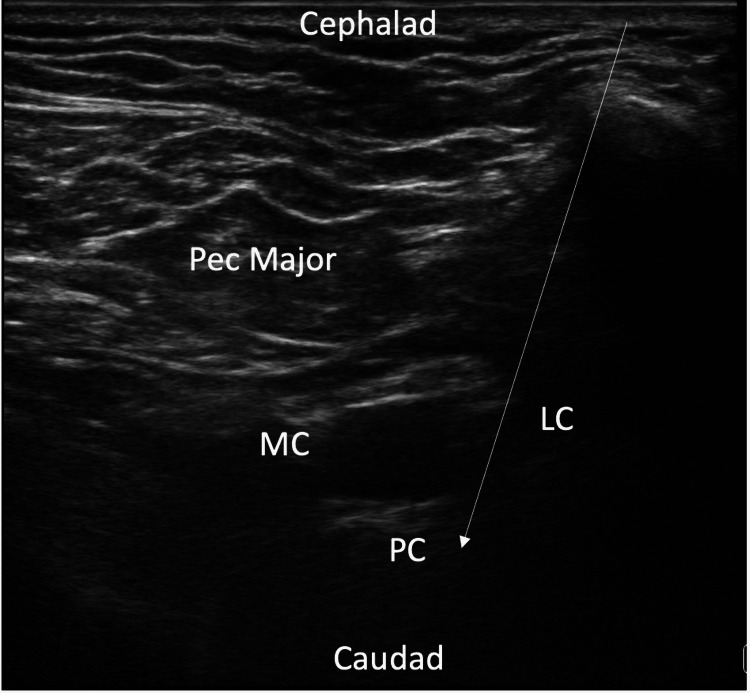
Ultrasound image of brachial plexus cords at the infraclavicular level and needle trajectory for the in-plane block technique. MC: medial cord; LC: lateral cord; PC: posterior cord
